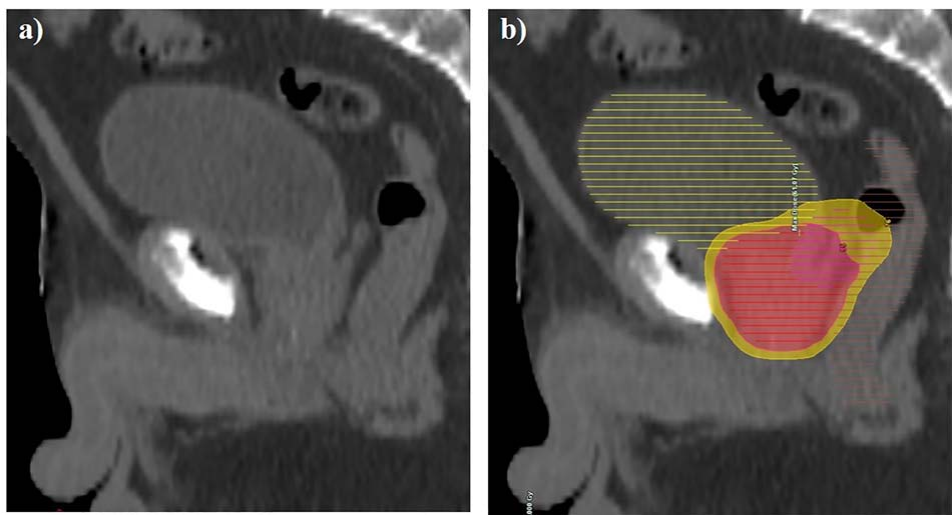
Sagittal external beam radiotherapy computed tomography planning scan performed at 6 months following the commencement of ADT (a–b). No suspicious mass protruding into the urinary bladder was convincingly visible on anatomical imaging (a). The patient received intensity-modulated radiation therapy using 60 Gy Prostate (red 95% isodose) and 48Gy seminal vesicles (yellow 95% isodose) delivered over 20 sessions with daily image guidance (b).
